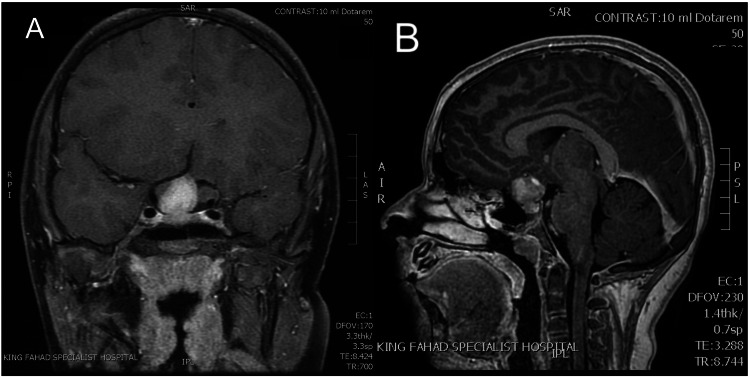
(A) Coronal (B) Sagittal T1-weighted contrast-enhanced MRI
